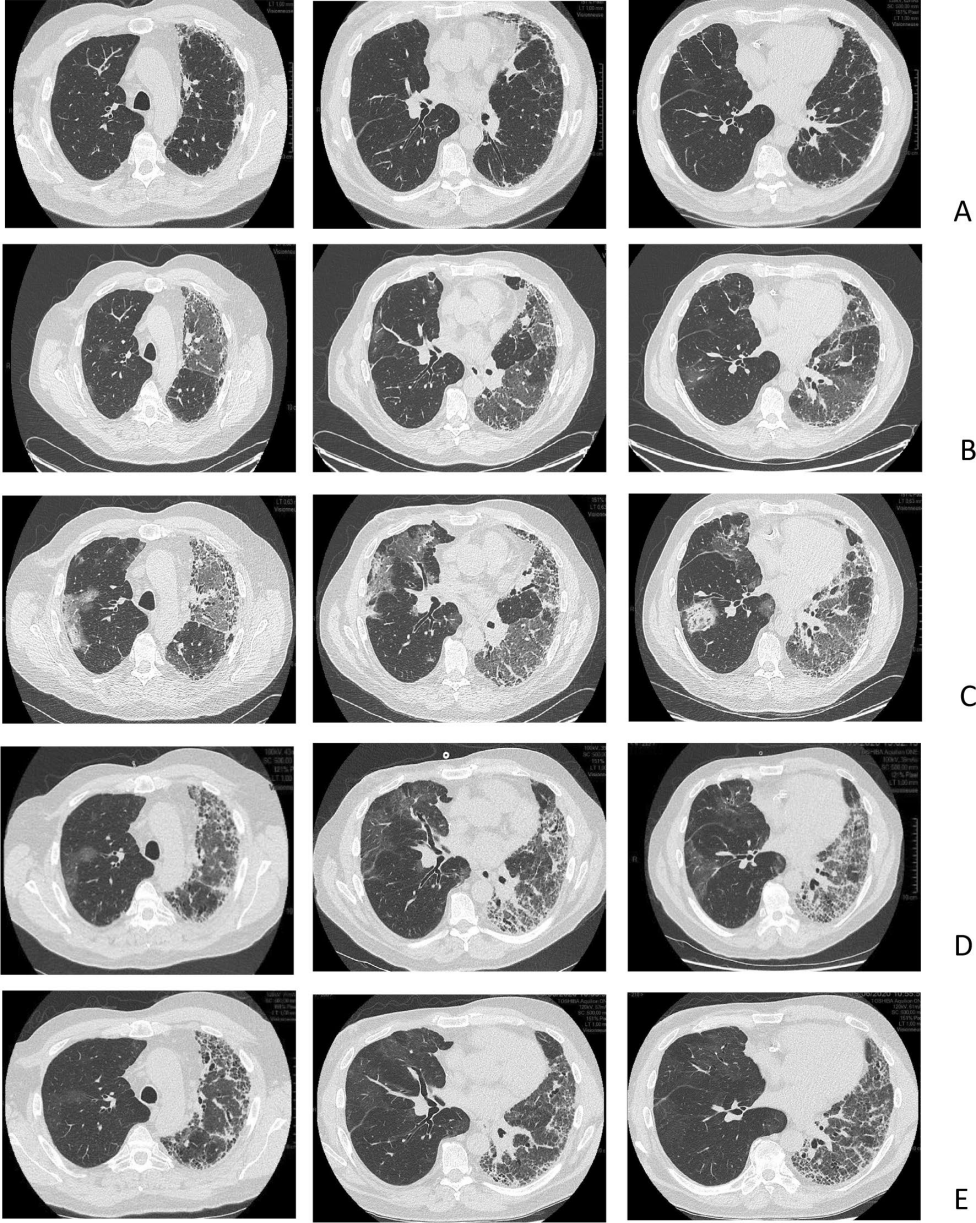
CT of the thorax in patient 2, a 62-year-old man, at different times after right SLT for IPF. A Postoperative day (POD) 90, before SARS-CoV-2 infection. B POD 104, when SARS-CoV-2 infection was diagnosed. C POD 110, at the time of transfer to the ICU for ARF. D POD 135, after discharge from the ICU. E POD 170 after discharge from the hospital.
